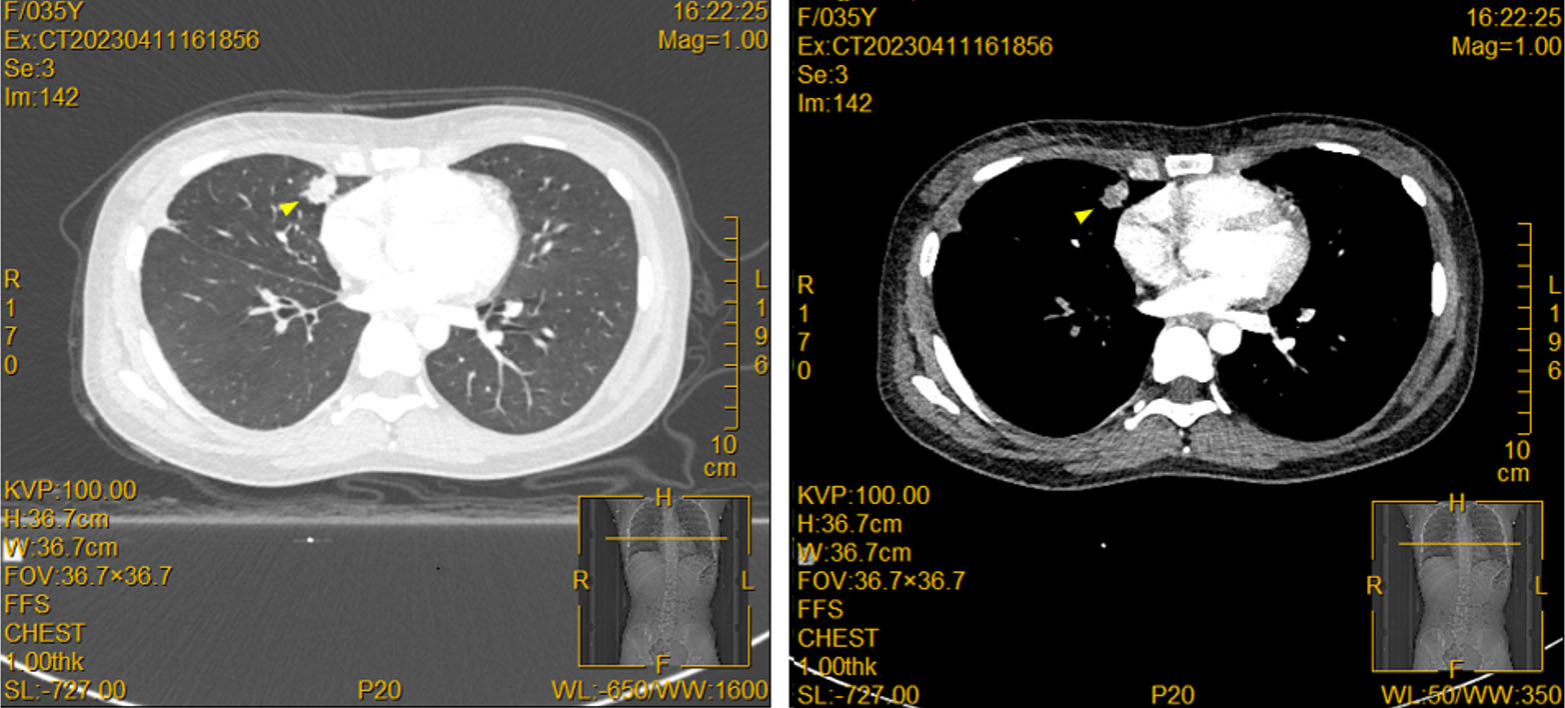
Pulmonary lesions are displayed in the contrast-enhanced CT. A solid nodule (yellow triangle) was observed in the inner segment of the right middle lobe (Img120), measuring approximately 16.6mm × 12.1mm, with lobulated and spiculated edges, and showing heterogeneous enhancement on contrast imaging.
